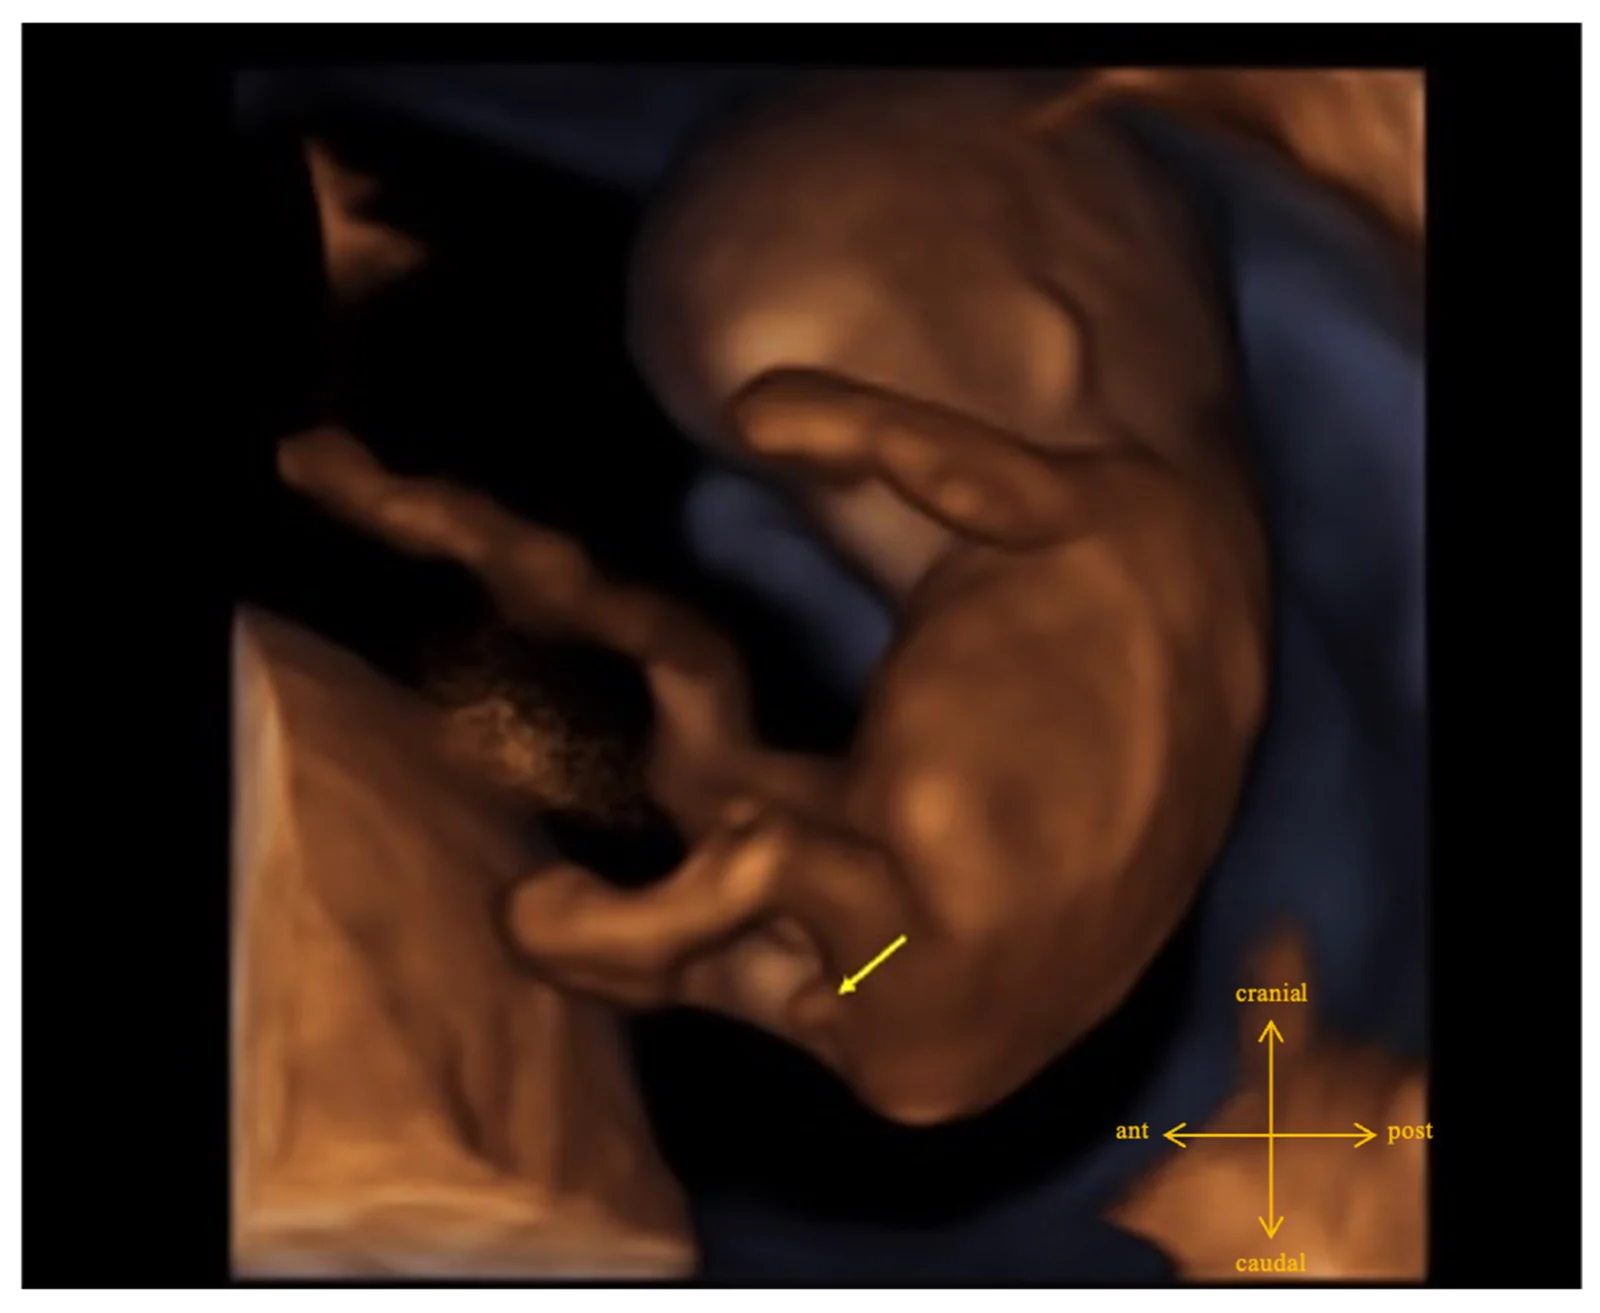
Ultrasonographic evaluation of the genital tubercle in an 8-week female fetus—the genital tubercle (yellow arrow) presents a caudal orientation, suggesting female sex.
